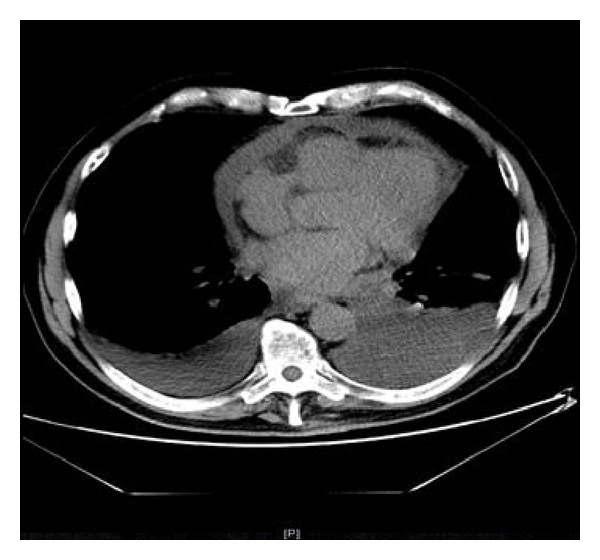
CT scan of chest with Pleuropericardial effusion. CT scan showing large Pleuropericardial effusion.
